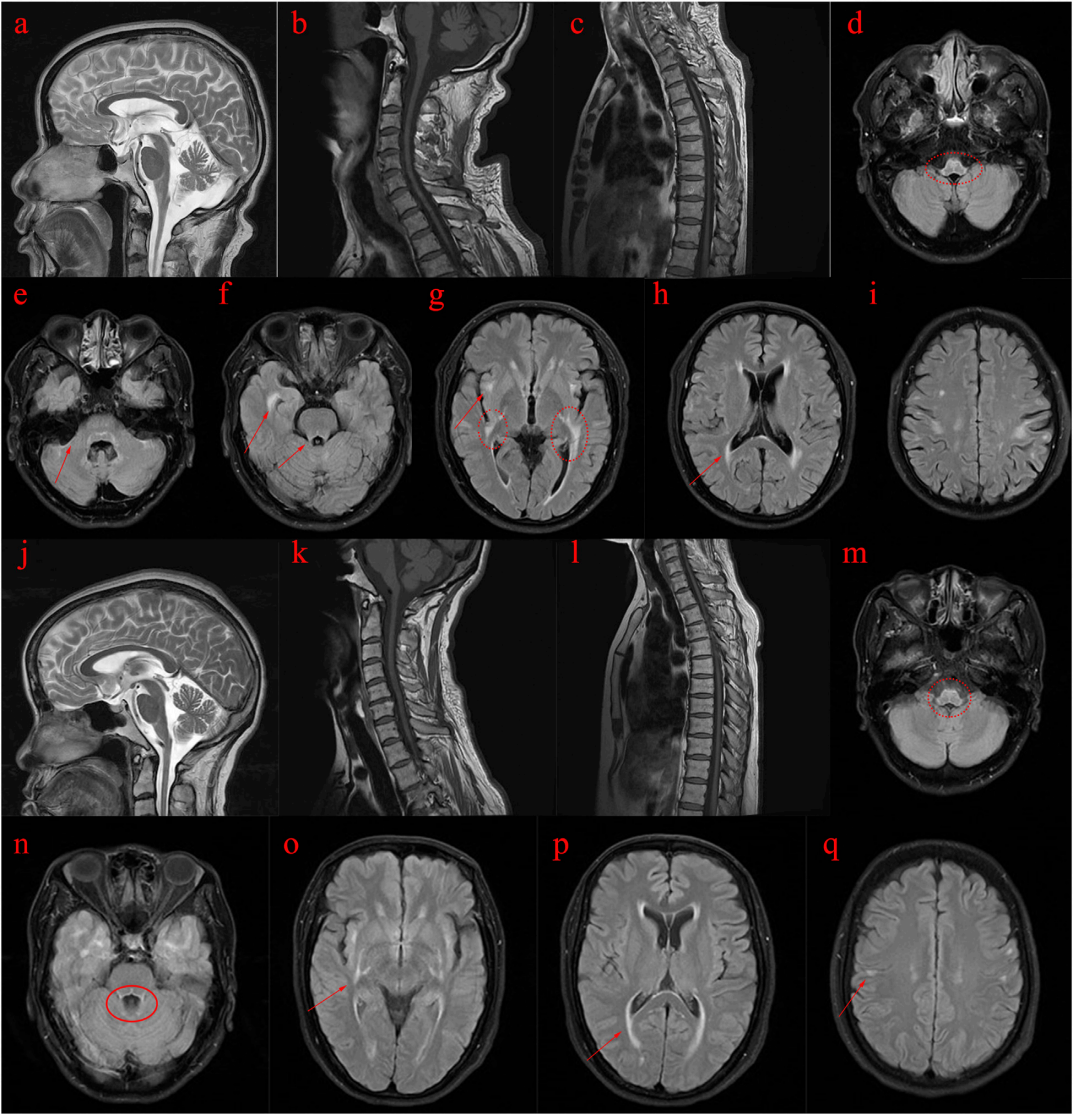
MRI images of the proband (II3) and his sister (II5). (A–I): MRI scans of the proband (Ⅱ3) reveal abnormal spinal cord and brain structures. (J–Q): MRI scans of the proband’s sister (Ⅱ5) also indicate abnormal spinal cord and brain structures. (A–C): T2-weighted images show atrophy of the cerebral cortex and cerebellum, while T1-weighted images depict pronounced atrophy in the cervical and thoracic spinal cord. (D–I): T2 FLAIR images demonstrate abnormal high signal intensity in the white matter with a symmetrical pattern, primarily affecting the pyramidal tract, periventricular regions, temporal lobe, posterior limbs of the external capsule, internal capsule, and surrounding the brainstem. (J–L): T2-weighted images of the proband’s sister reveal cerebral and cerebellar atrophy, along with significant atrophy of the cervical and thoracic spinal cord on T1-weighted images. (M–Q): T2 FLAIR images show abnormal high signal intensity in the white matter, symmetrically affecting regions similar to those in the proband, including the pyramidal tract, periventricular area, temporal lobe, external and internal capsule, and around the brainstem.
